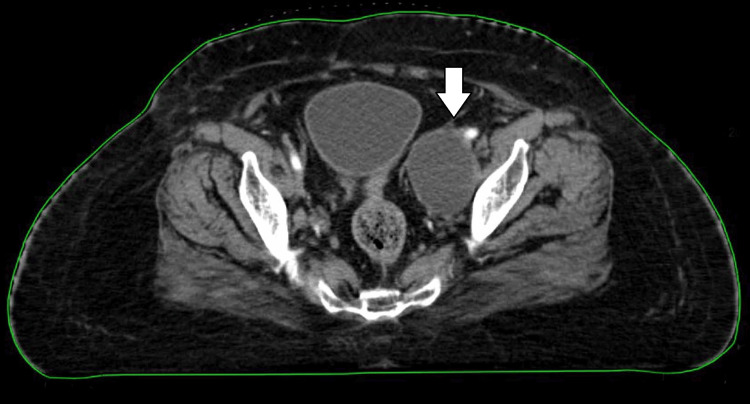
Planning CT showing large lymphocele at the pelvic region on the left side near the left external iliac vessel CT: computed tomography
